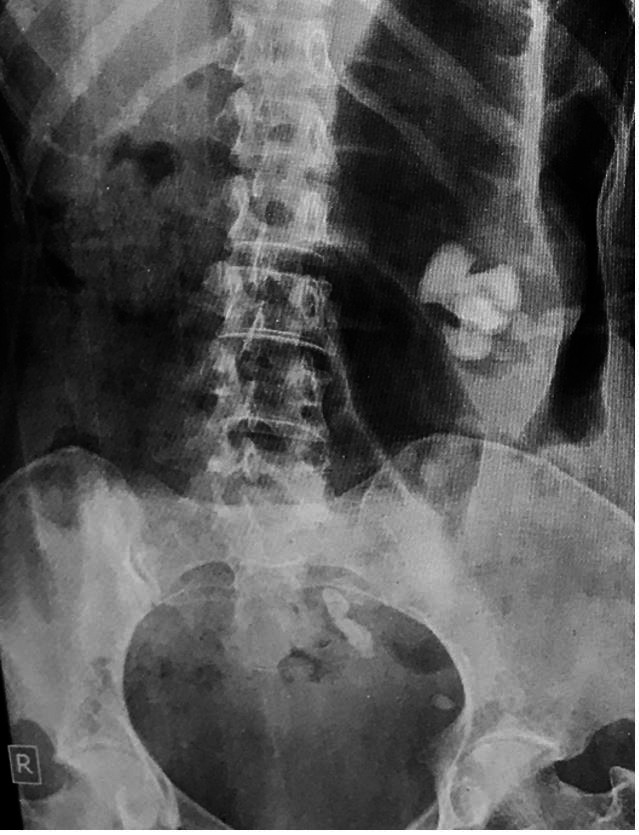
Abdominal plain radiograph shows a large radio-opaque shadow in the left renal fossa and multiple small radio-opaque shadows in the left pelvic region. These findings are consistent with multiple calculi.
